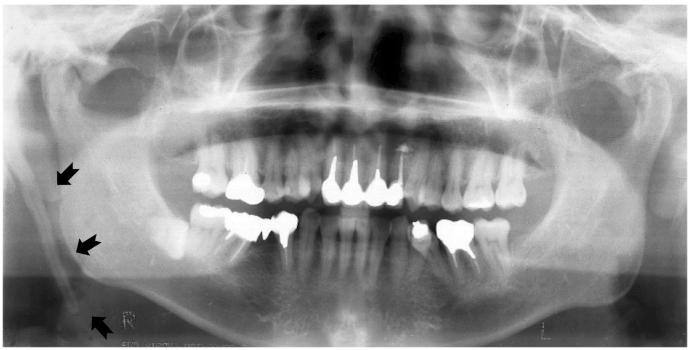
Panoramic radiology of the initial visit. Prolongation of the styloid process beyond the mandibular plane was observed (arrows).
